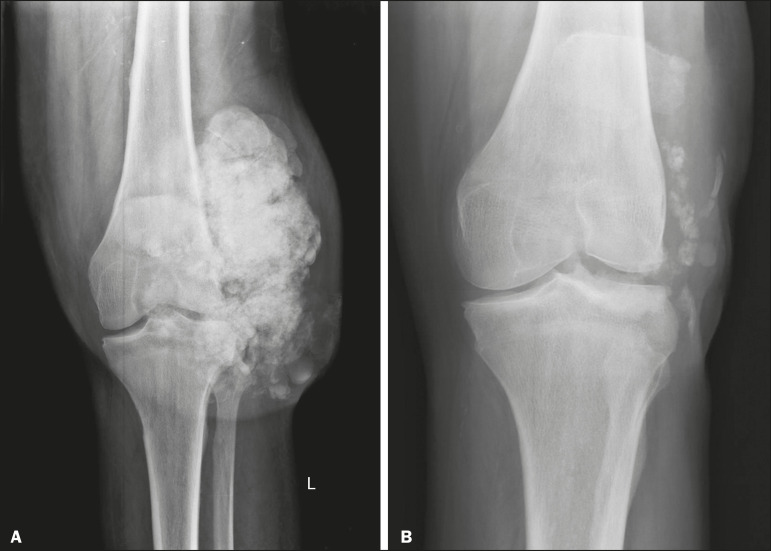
Metastatic calcifications in end-stage renal disease. A: Frontal X-ray of the knee showing a periarticular calcified mass with lobulated contours on the lateral face of the knee, with a multicystic appearance and a fluid-calcium level (the sedimentation sign). Note that the radiological appearance is identical to that of tumoral calcinosis. B: Frontal X-ray of the knee two years after kidney transplantation showing significant shrinkage of the lesions.
